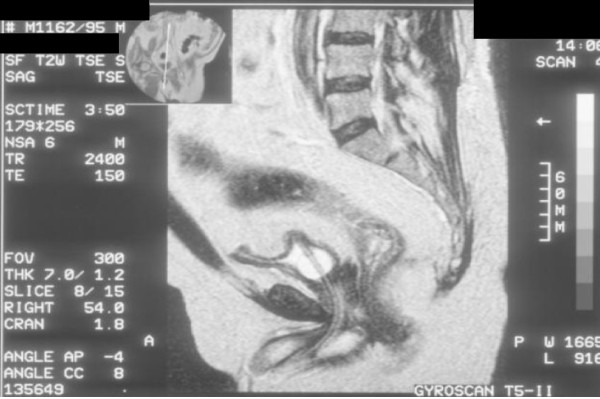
Magnetic Resonance Imaging of pelvis performed on 23 October 1995: Sagittal T-2 weighted image shows urinary catheter in urethra. There is no extra-capsular spread of carcinoma of prostate.
